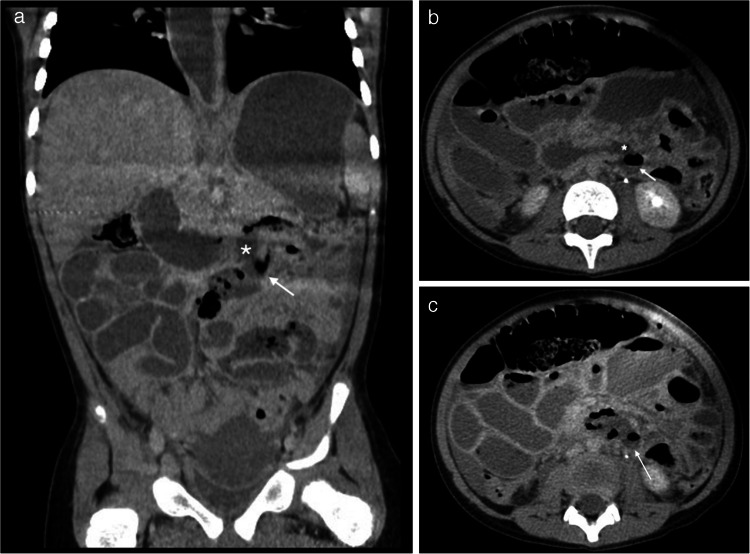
A 3-year-old male presented with a rigid abdomen after a reported low-height fall. CT of the abdomen and pelvis with only IV contrast using split-bolus technique revealed duodenal perforation that was subsequently confirmed at surgery. Findings elicited concern for physical abuse. a Coronal image shows a focal disruption of the inferior wall of the distal duodenum (*white asterisk*) which communicates with a collection containing extraluminal air and fluid (*white arrow*). b Axial image shows a focal disruption of the posterior wall of the distal duodenum (*white asterisk*) which communicates with the retroperitoneal collection containing fluid and extraluminal air (*white arrow*). c Axial image at a more caudal location better delineates a sizable retroperitoneal collection containing fluid and extraluminal air (*white arrow*) exerting mass effect on the adjacent bowel which is displaced anteriorly
